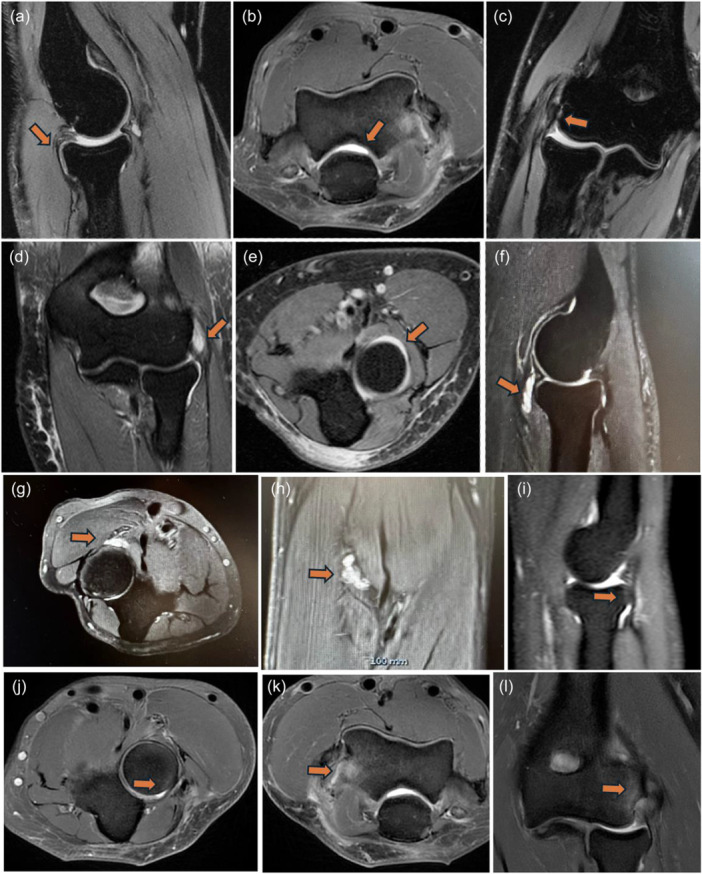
Examples of findings evaluated on the preoperative MRI. (a) Annular ligament sagging (arrow) in a sagittal PD FS image. (b) Lateral minor incongruency (asymmetry) of the ulnohumeral joint (arrow) in an axial PD FS image. (c) R‐LCL proximal tear/discontinuity—L‐sign (arrow) in a coronal PD FS image; the synovial fluid accumulated between the radial collateral ligament (R‐LCL) and the capitellum creates the vertical line, while the radio‐capitellar joint space forms the horizontal line—together resembling the shape of an ‘L; (d) Common extensor partial tear (arrow) in a coronal PD FS image. (e) Annular ligament sagging/fluid around the RH‐Eclipse sign (arrow) in an axial PD FS image. (f) Anterior peri‐annular synovial cyst (arrow) in a sagittal PD FS image. (g) Anterior peri‐annular synovial cyst (arrow) in an axial PD FS image. (h) Anterior peri‐annular synovial cyst (arrow) in a coronal PD FS image. (i) Radial head chondropathy (arrow) in a sagittal PD FS image. (j) Radial head chondropathy (arrow) in an axial PD FS image. (k) Capitellum lateral chondropathy (arrow) in an axial PD FS image. (l) Capitellum lateral chondropathy (arrow) in a coronal PD FS image. FS, fat‐saturated; MRI, magnetic resonance imaging; PD, proton density.
